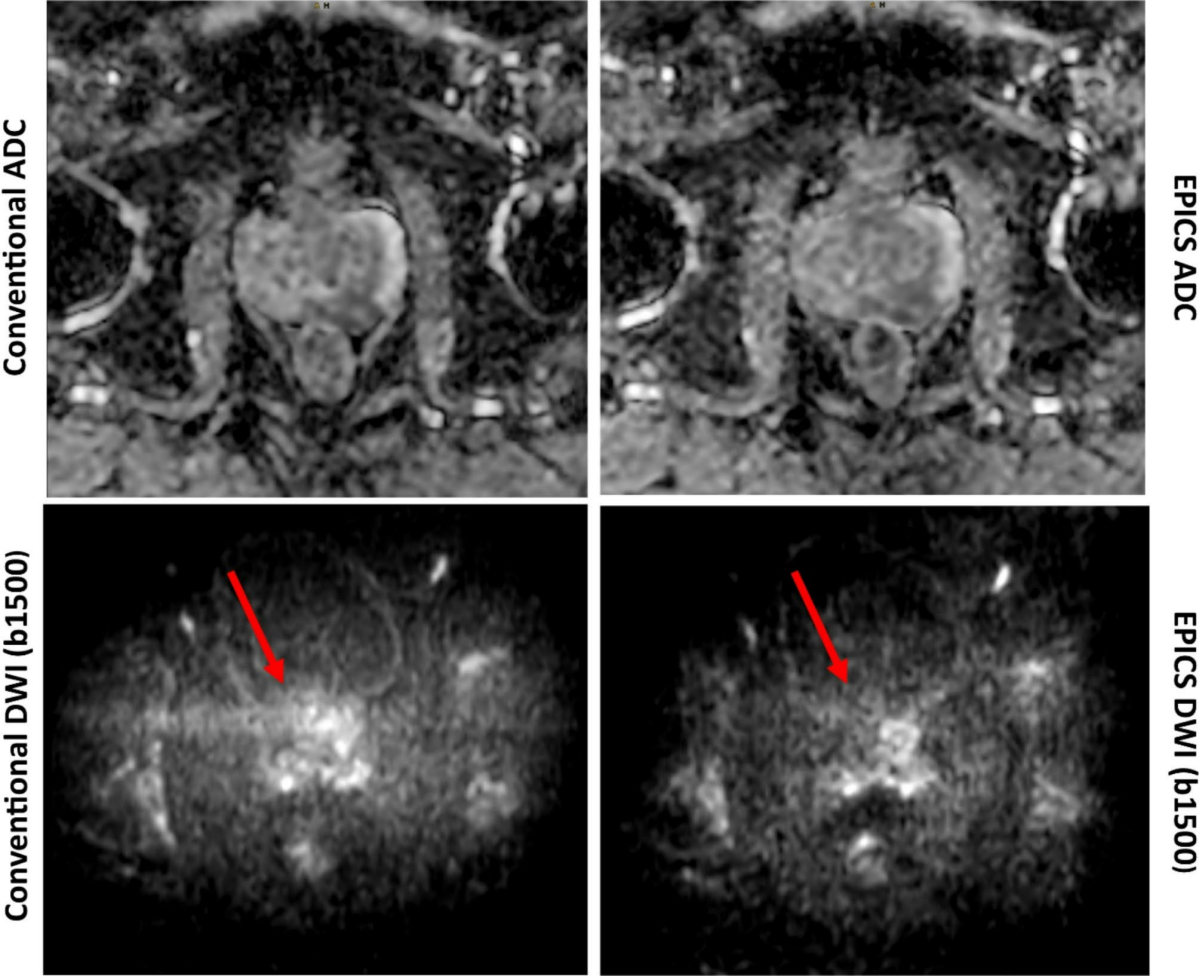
DWI b1500 images and apparent diffusion coefficient (ADC) maps obtained from conventional diffusion-weighted images (DWI) and accelerated DWI sequences using echo planar imaging and compressed SENSE based reconstruction (EPICS). In this example artifacts (arrows) are reduced in EPICS DWI (b1500) compared to conventional reconstruction. No significant changes are seen in the ADC maps.
